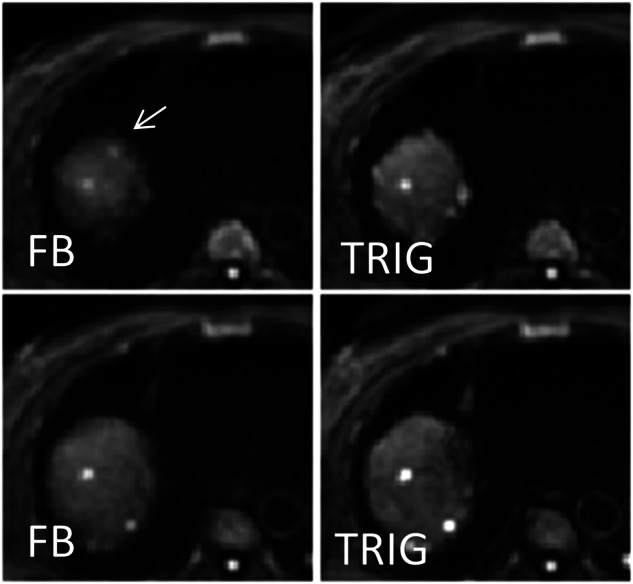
Liver DWI b800 images of a 79-year-old patient with metastatic neuroendocrine tumor. Two adjacent slices are shown for FB (left) and TRIG (right). The lesion is only visible in FB and is marked with a thin arrow. Other lesions are recognizable in neighboring layers. FB, free-breathing acquisition; TRIG, navigator-triggered acquisition
